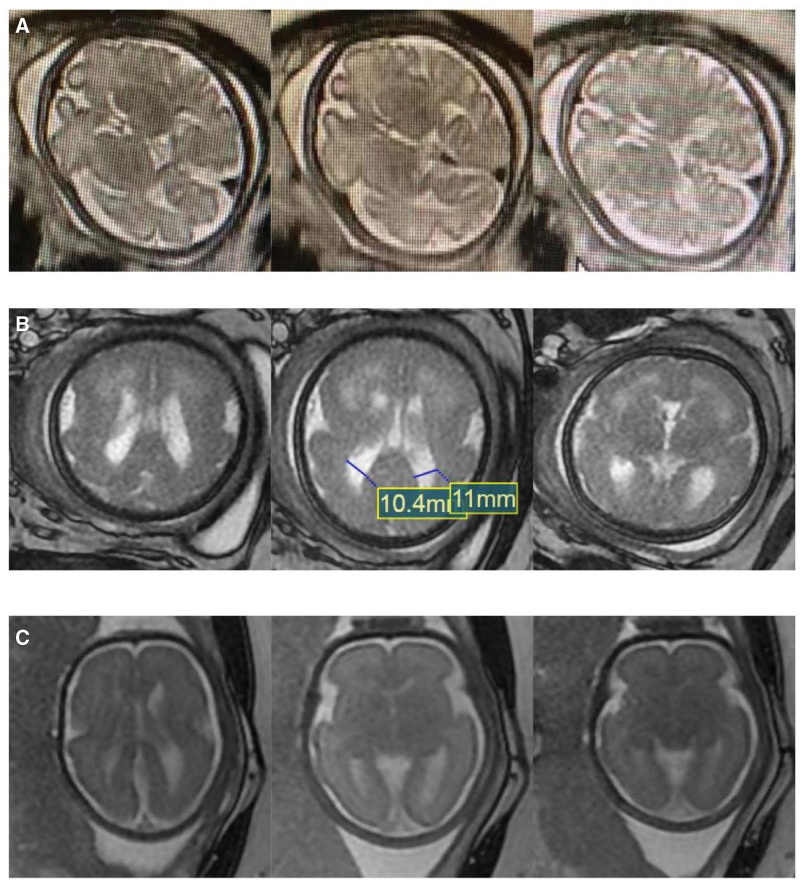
Prenatal fetal MRI findings. (A) Thinning of the white matter in the temporal and frontal parietal areas of both sides of the brain, as well as patchy shadows under the frontal lobe cortex on both sides. Compared to a normal fetus at 37 wks of gestation, it was determined that the white matter in the brain of the fetus was dysplastic. (B) The brain contour exhibited the “8” character sign, and a curved T2 high signal shadow was observed in the frontal parietal cortex. The width of the posterior horn of the left and right ventricles was approximately 11 and 10.4 cm, respectively, with a transparent compartment cavity present. No obvious widening was observed in the cisterna magna, and the cerebellar vermis was visible. (C) A normal signal. MRI = magnetic resonance imaging.
